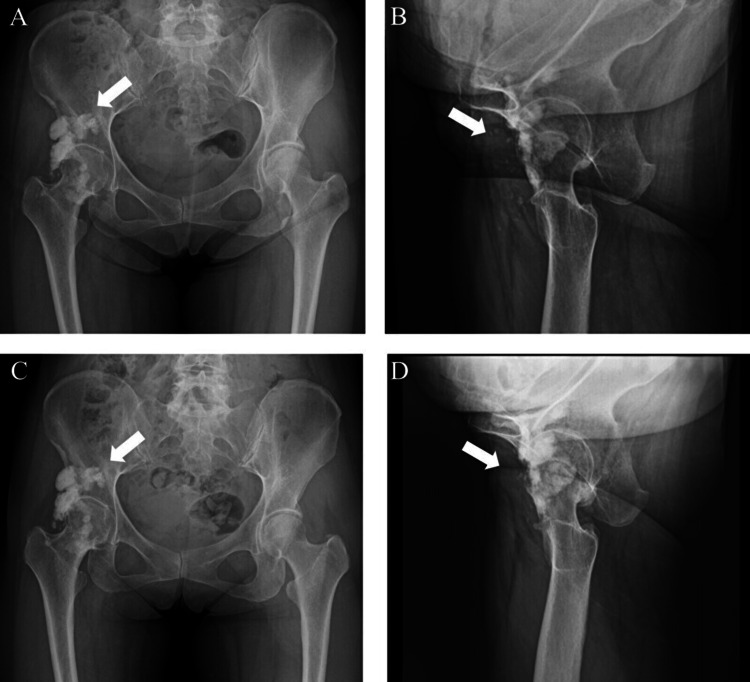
Postoperative plain radiographs of the right hip in anteroposterior position (A) and lateral position (B). Plain radiographs at last follow-up in anteroposterior position (C) and lateral position (D). No apparent regrowth was observed on the radiograph at the final follow-up.
